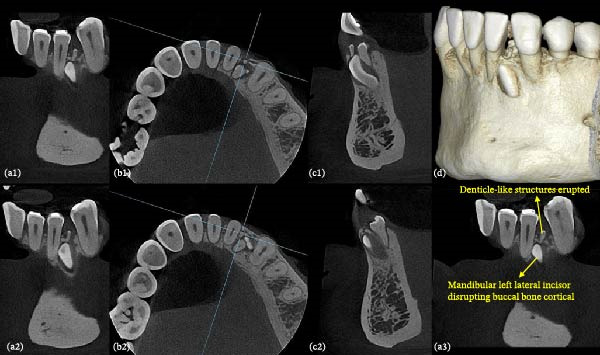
CBCT assessment. (a1, a2, a3) Coronal, (b1, b2) axial, and (c1, c2) sagittal planes, as well as (d) a three‐dimensional reconstruction, showing the spatial relation between the embedded lateral incisor, the supernumerary tooth, and the denticle‐like structures compatible with a compound odontoma. Cortical bone disruption at the alveolar ridge supports the suspicion of a partially erupted intraosseous compound odontoma.
